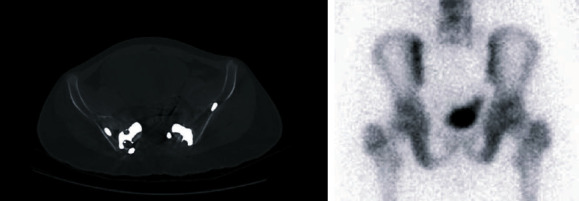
Interface of bone-implant by the CT scan. CT scan during follow-up at 12 months in the patient (patient no. 3) with sacral Ewing's sarcoma. No radiolucence was observed at bone-implant interface. Also, the bone scan showed higher bone metabolism at the interface, which may be caused by the compression provided by the fixation screws.
